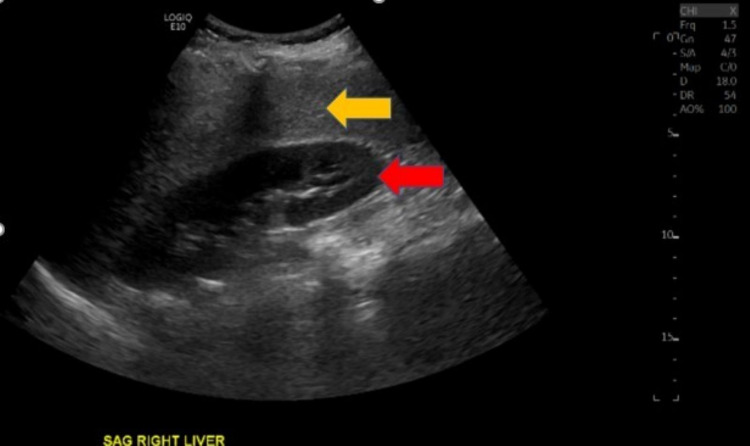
Ultrasound imaging of left liver lobe (sagittal plane) Ultrasound imaging (sagittal plane) of right liver lobe parenchyma (yellow arrow) adjacent to right kidney (red arrow) demonstrating hepatic steatosis.
